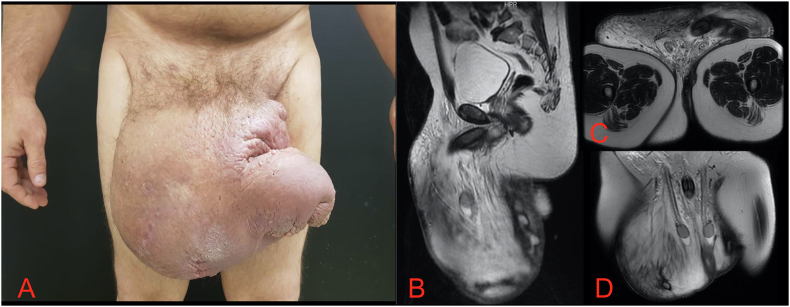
A: Clinical presentation of giant penoscrotal lymphedema; B: Sagittal section of MRI; C: Axial section of MRI; D: Coronal view of MRI with increased testicular pouch volume, diffuse subcutaneous edema and scrotal wall thickening.
